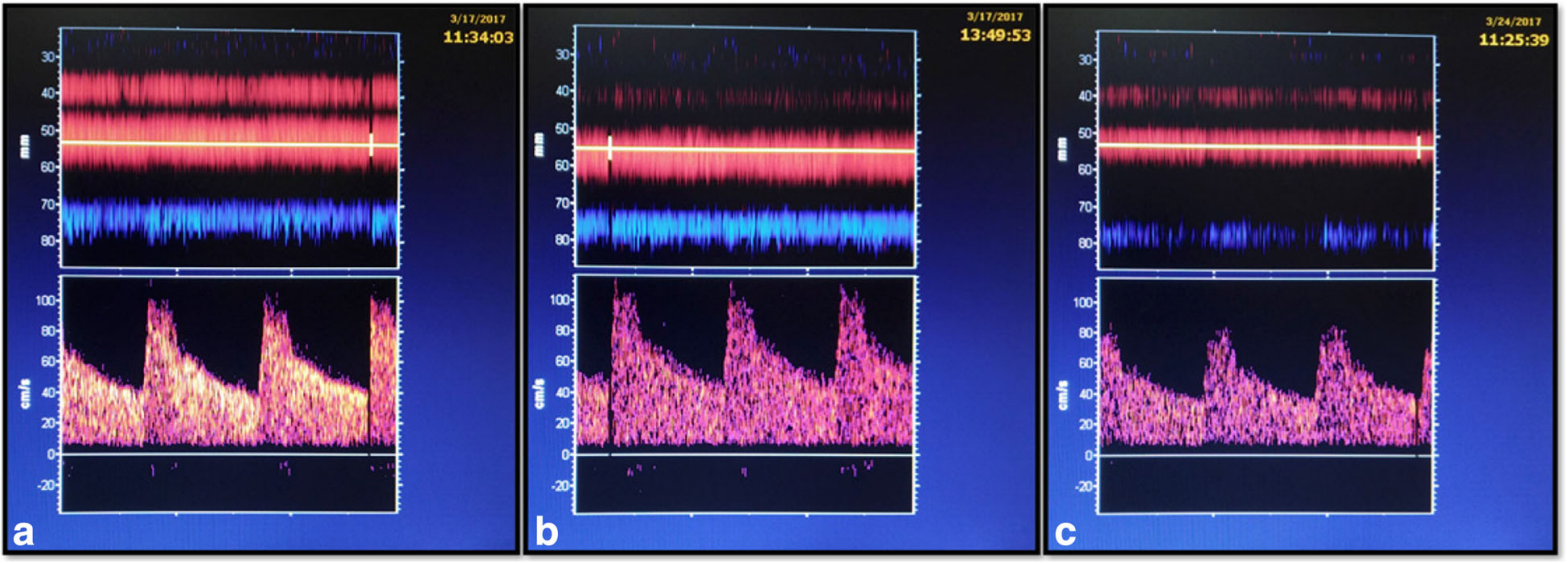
Three transcranial Doppler examinations of the same participant (visit 1: images a and b; visit 2: image c)
